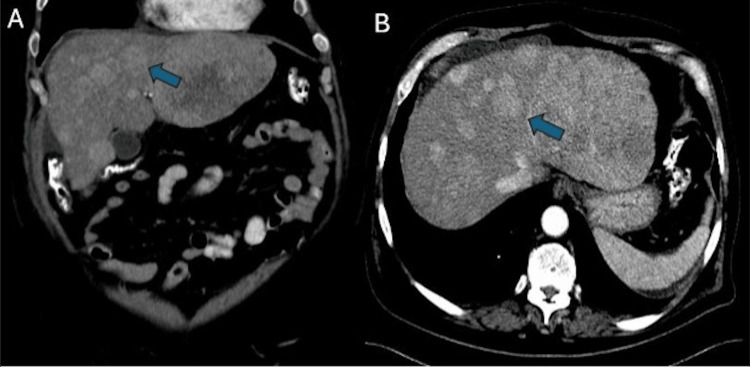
CT scan of the abdominal cavity showing widely spread heterogenous metastasis of the neoplasm to the liver (blue arrows). A) Coronal plane CT scan with contrast; B) transverse plane CT scan with contrast
